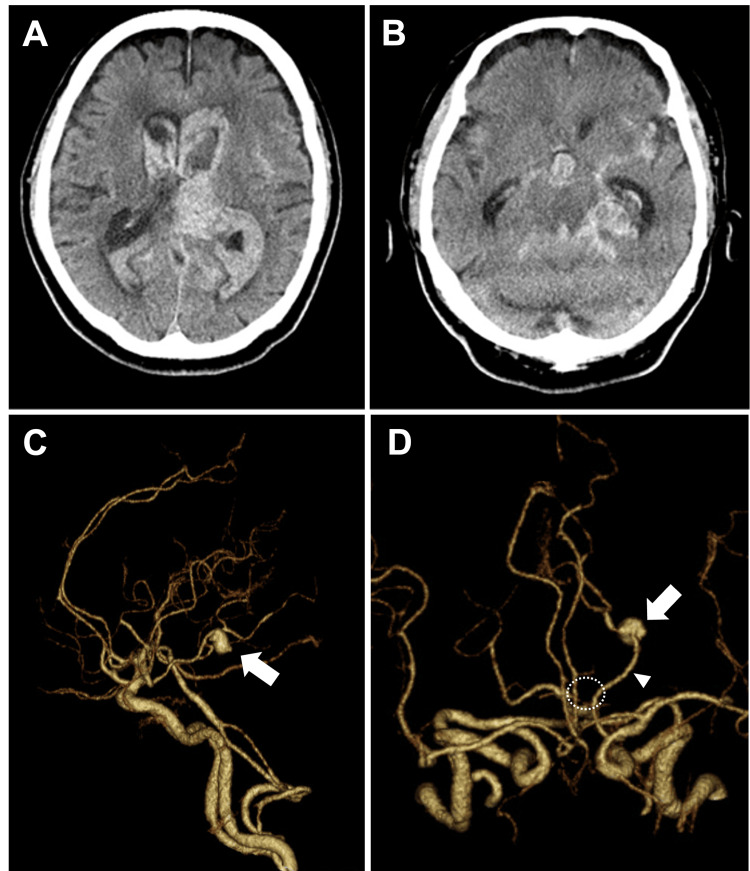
Initial CT findings of the ruptured posterior cerebral artery aneurysm (A, B) Axial non-contrast head CT images obtained on the day of onset show diffuse subarachnoid hemorrhage (SAH), with a particularly thick clot in the left ambient cistern. Acute obstructive hydrocephalus is also observed. (C) Lateral and (D) superior views of contrast-enhanced head CT demonstrate a ruptured aneurysm, considered the source of bleeding, located in the distal P2 segment of the left posterior cerebral artery (PCA) (white arrow). The left posterior communicating artery (PCoA) is the fetal-type (white arrowhead), and the ipsilateral P1 segment of the PCA is hypoplastic (dashed circle). CT: computed tomography
